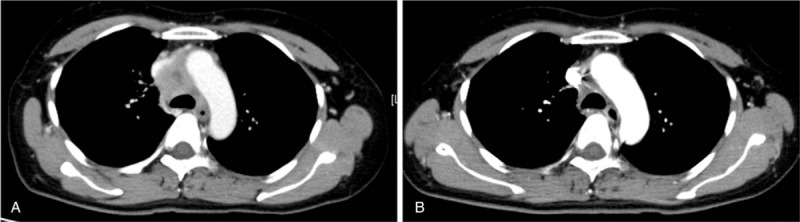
Chest-enhanced CT images; (A) Before pembrolizumab administration (B) After 3 courses of administration. CT = computed tomography.
